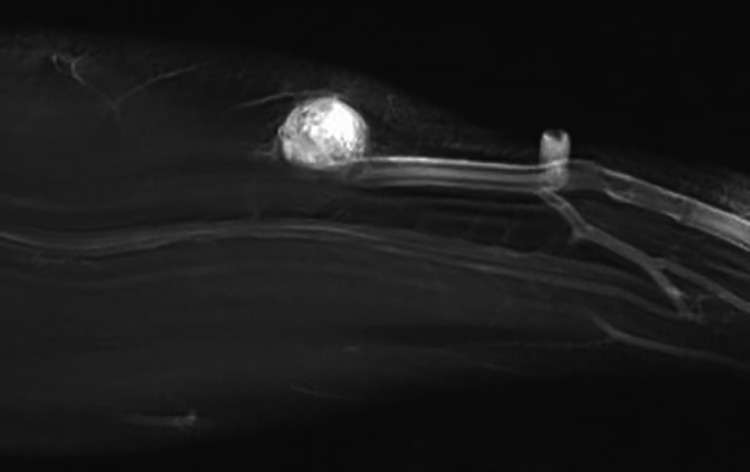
MRI of left forearm, sagittal view. T1-weighted image with contrast shows contrast-enhancing mass compressing the superficial branch of the radial nerve and associated vascular bundle.
